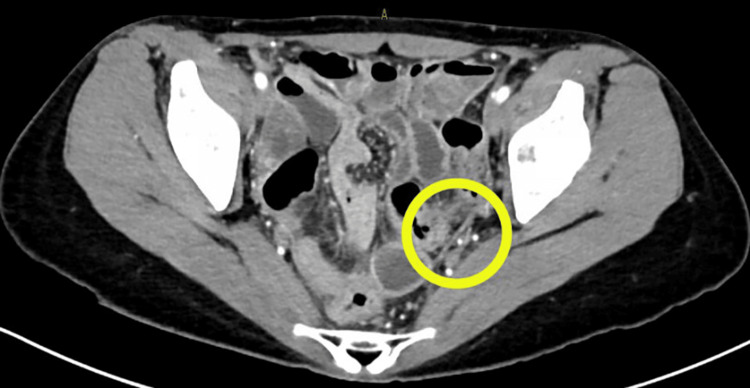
Pelvic computed tomography (CT) with contrast
